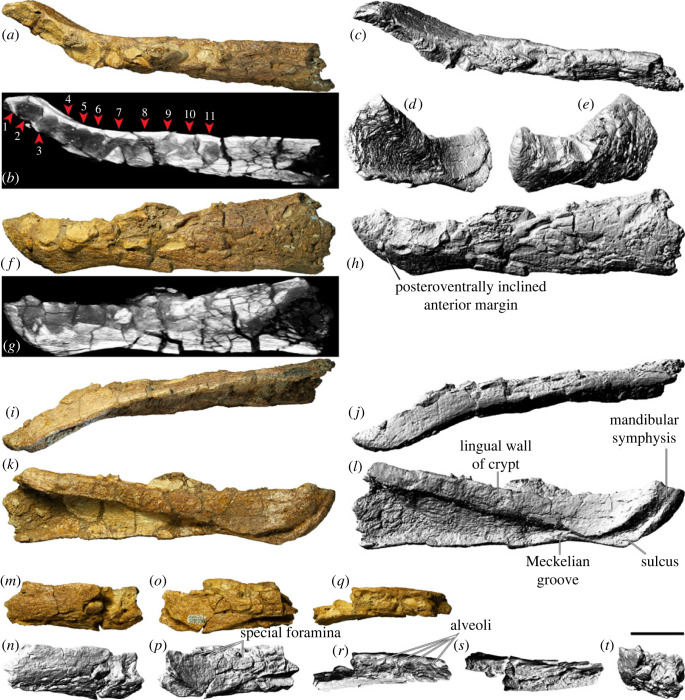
Diamantinasaurus matildae* referred dentaries (AODF 0906). (*a–l*) Left dentary in (*a–c*) occlusal, (*d*) posterior, (*e*) anterior, (*f–h*) lateral, (*i–j*) ventral and (*k–l*) medial views. (*a*), (*f*), (*i*) and (*k*) are photographs; (*b*) and (*g*) are three-dimensional models derived from CT scans; and (*c–e*), (*h*), (*j*) and (*l*) are three-dimensional models derived from surface scans. Numbers 1–11 indicate alveoli. (*m–t*) Right dentary in (*m–n*) lateral, (*o–p*) medial, (*q–r*) occlusal, (*s*) ventral and (*t*) posterior views. (*m*), (*o*) and (*q*) are photographs, (*n*), (*p*) and (*r–t*) are three-dimensional models derived from surface scans. Scale bar = 50 mm.
